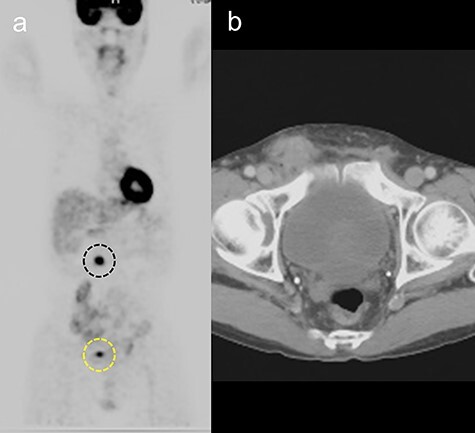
(a) Fluorine-18 fluorodeoxyglucose positron emission tomographic scan showing positive findings in the transverse colon (black dotted line) and right inguinal area (yellow dotted line). (b) Computed tomography scan showing a mass with indistinct margins in the right inguinal area, 5 years after hernia repair.
